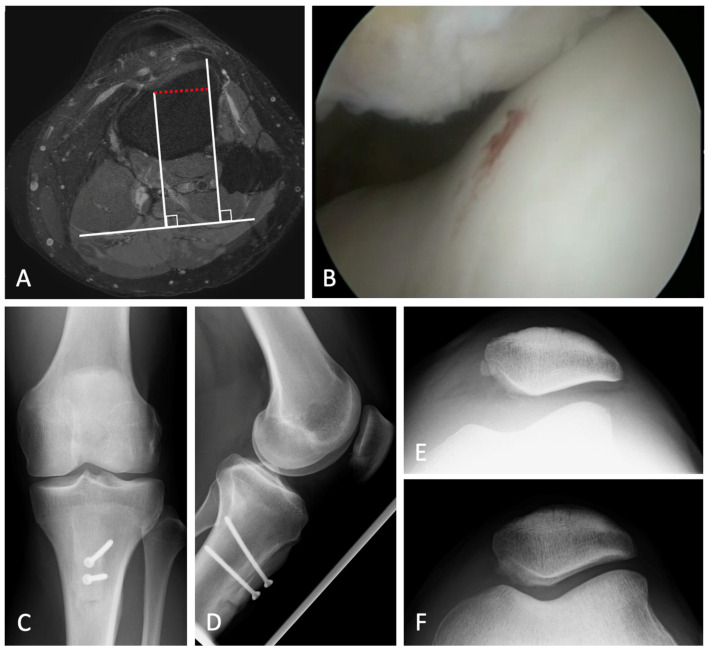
Lateral patellar maltracking in a left knee. Preoperative analysis revealed a TT-TG (tibial tubercle to trochlear groove) distance of 18 mm, with no further risk factors (A). Intraoperative arthroscopy (with low water pressure) confirmed a lateralized patellar tracking (B), reinforcing the indication to perform a medializing osteotomy of the tibial tubercle (C,D). A comparison of pre- and postoperative tangential radiographs of the patella showed a successful realignment of the patella (E,F).
